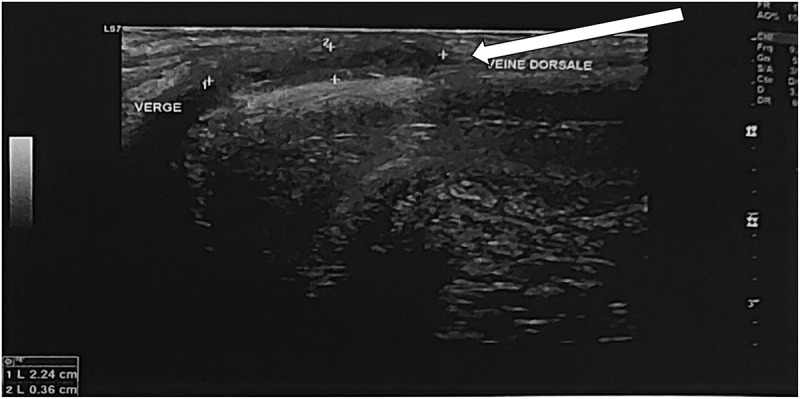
Ultrasound evaluation of the deep dorsal penile vein shows dilatation and endoluminal echogenic thrombosis measured at 3.6 mm and extended for 22 mm.
